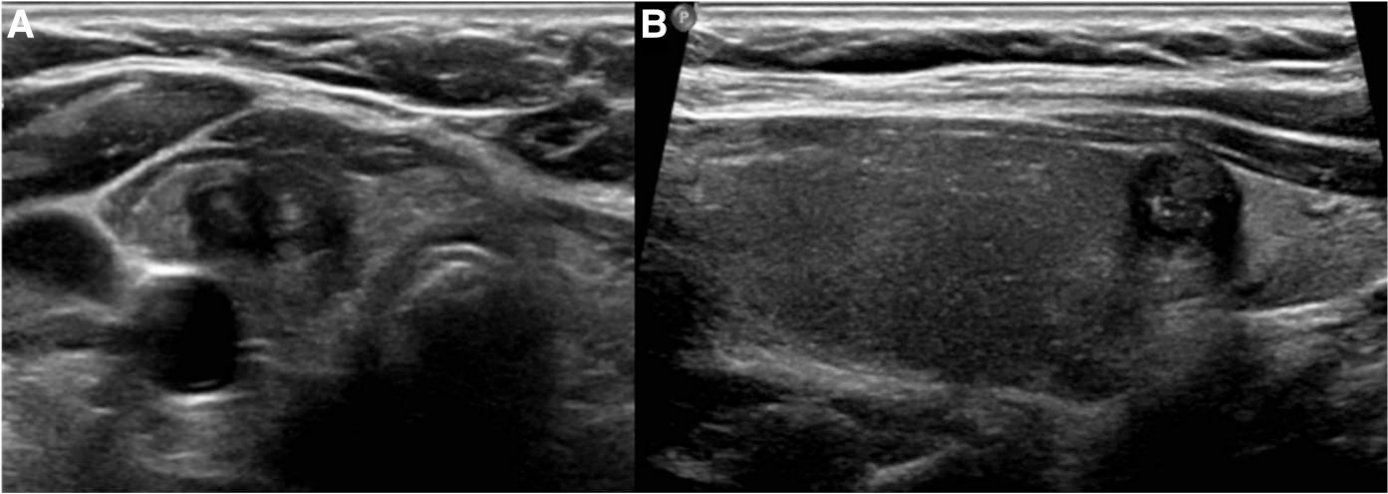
Thyroid ultrasound: A, transverse and B, sagittal views showing right lower pole 1.4 cm markedly hypoechoic nodule with irregular/lobulated margins and punctate echogenic foci (American College of Radiology Thyroid Imaging and Data System 5, high suspicion).
